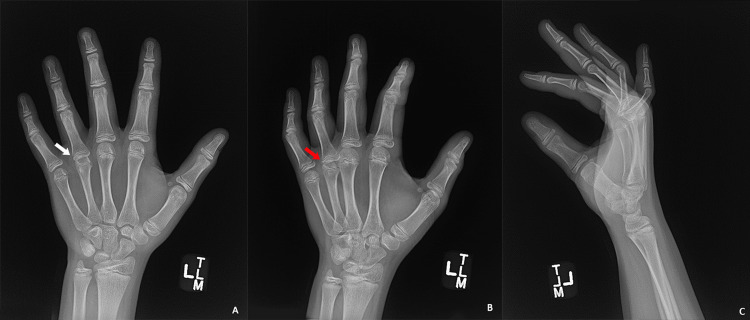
Plain film radiographs of the patient's left hand (a) Anterior-posterior view. Lucency and loss of height of the fourth metacarpal head (white arrow). (b) Oblique and (c) lateral views. Volar subluxation of the fourth MCP joint (red arrow) MCP: metacarpophalangeal
